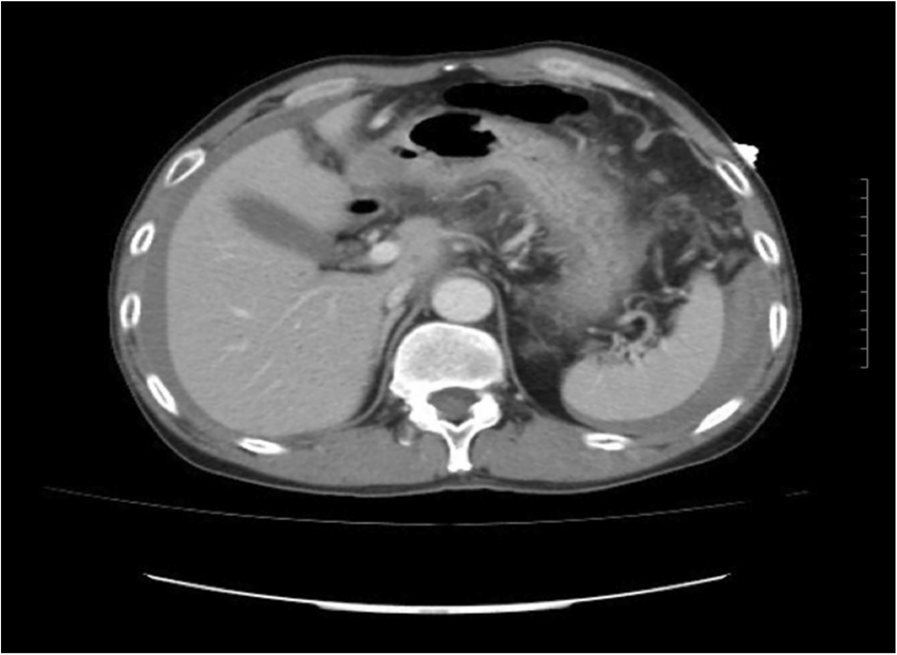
Abdominal CT scan. The CT-scan receals structural disorder of the left upper abdominal and hemoperitoneum
